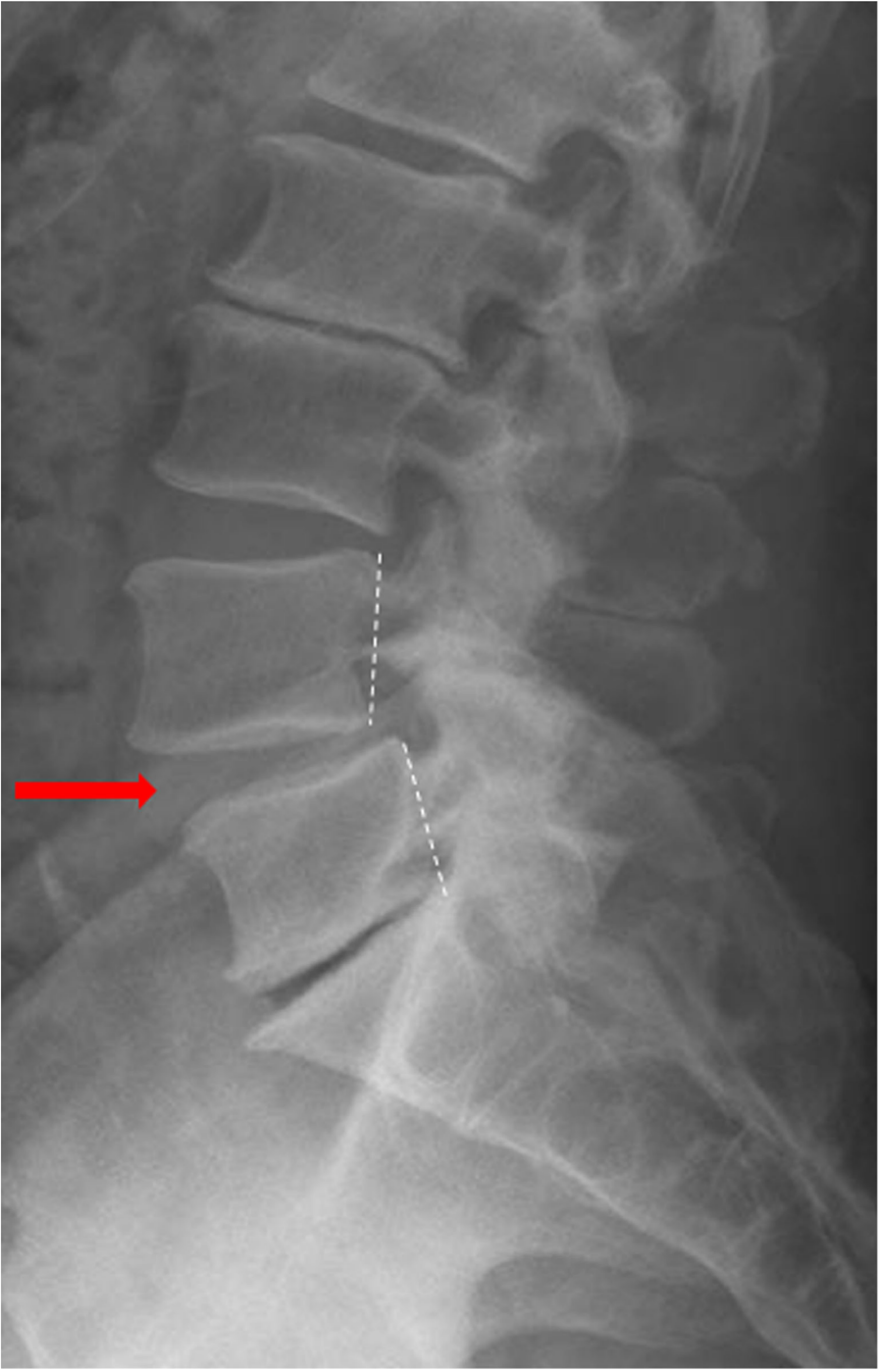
Lateral standing plain radiograph noting degenerative spondylolisthesis at L4–L5
